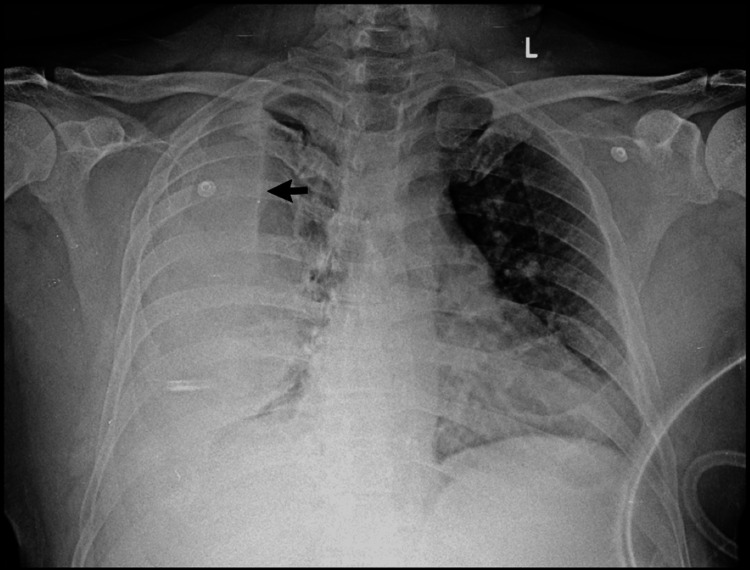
Pre-procedural chest radiograph showing effusion progression. Anteroposterior chest radiograph demonstrating progression of the massive right-sided pleural effusion (arrow) causing near-complete opacification of the right hemithorax and contralateral mediastinal shift, necessitating therapeutic thoracentesis.
